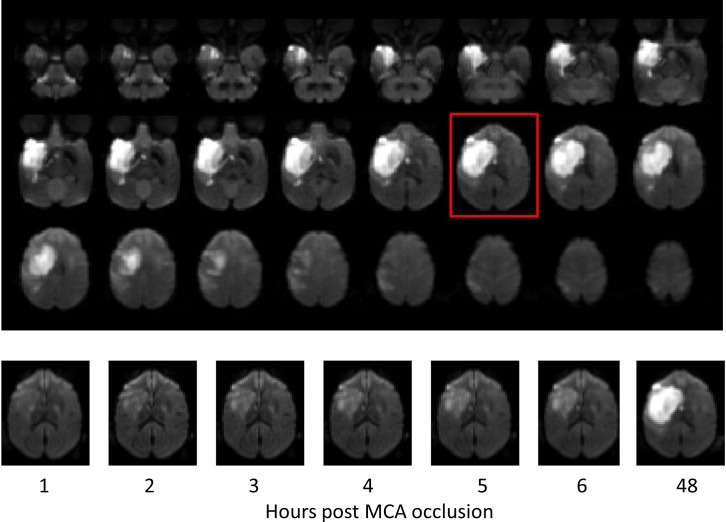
Stroke lesion evolution. Top: Axial DWI images of Subject RJJ3 at 48 hours post stroke show the entire infarct territory. Bottom: representative DWI images of RJJ3 demonstrate the infarct evolution at different time point (in hours) post MCA occlusion.
